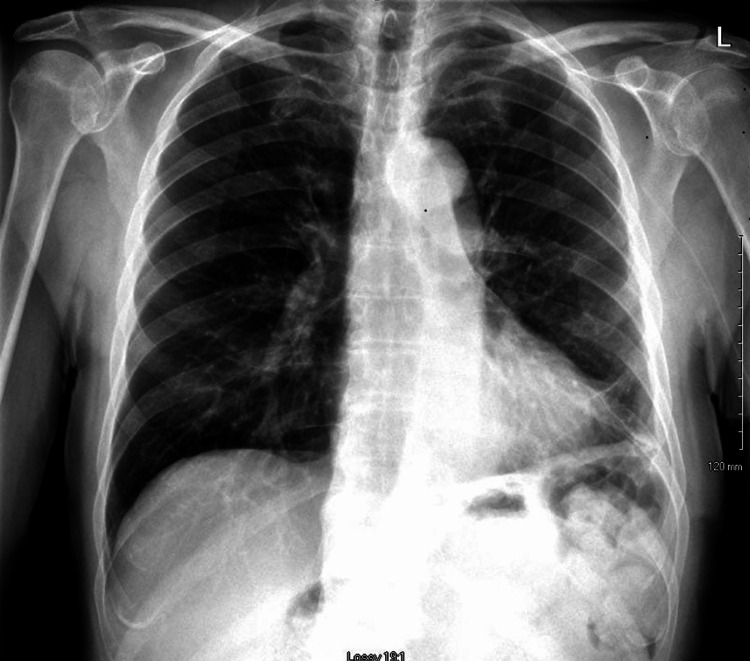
Post-Surgical Chest X-Ray A post-VATS chest X-ray showed the resolution of left-sided pleural effusion.
